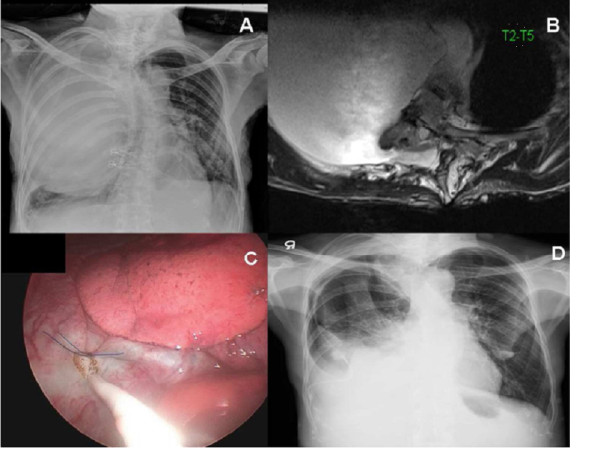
Chest plain radiograph findings before and after 3 months of thoracoscopic plication. (A) A large opaque shadow was seen in the right lung field with atelectasis of the lower lobe and kyphoscoliosis of thoracic spine was marked. (B) T2-weighted magnetic resonance imaging showed cerebrospinal fluid signal intensity and a meningeal cystic lesion protruding through the right neural foramen at T4. (C) The catheter was inserted into the meningocele, and the purse-string suture was tied under thoracoscopic guidance. (D) Postoperative chest radiograph at 3 months later showed marked regression of the intrathoracic meningocele.
